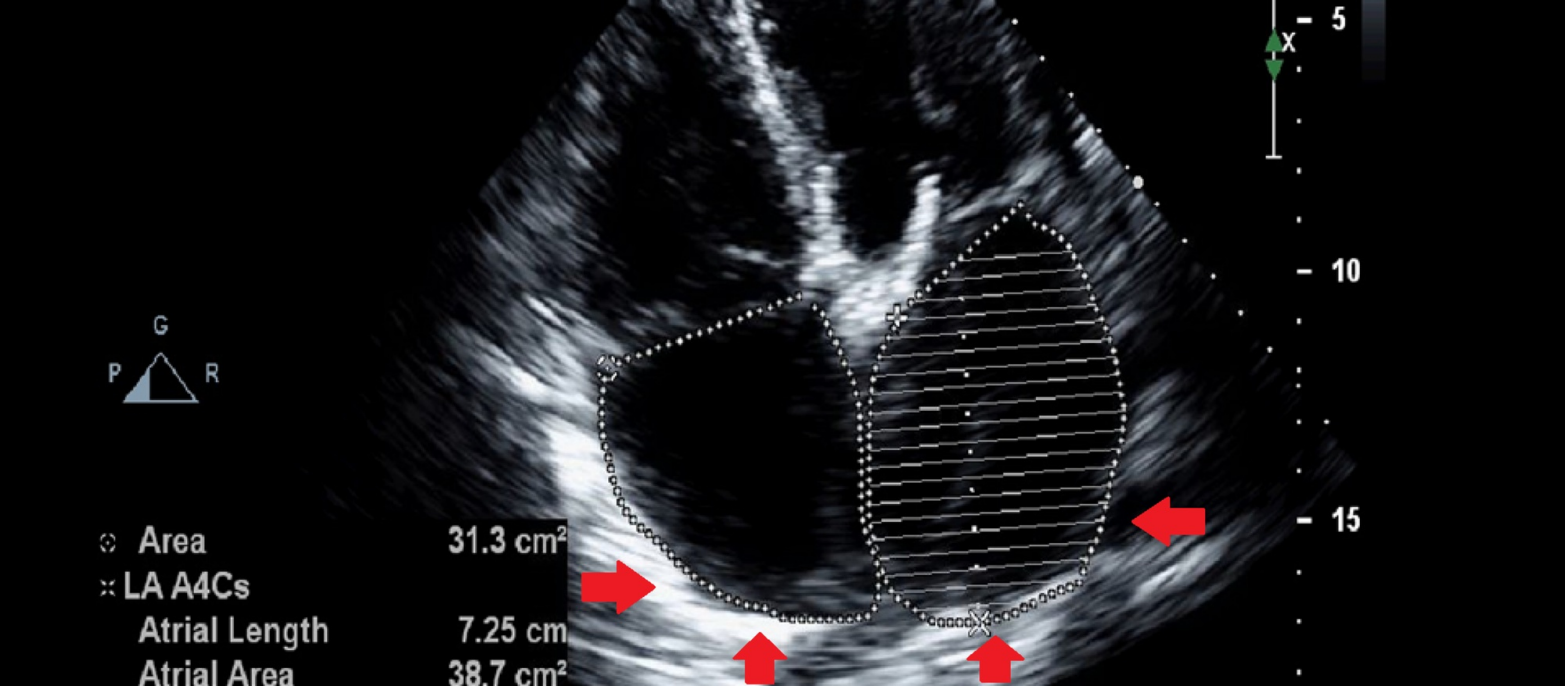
Transthoracic echocardiogram with an apical four-chamber view showing bi-atrial dilation
